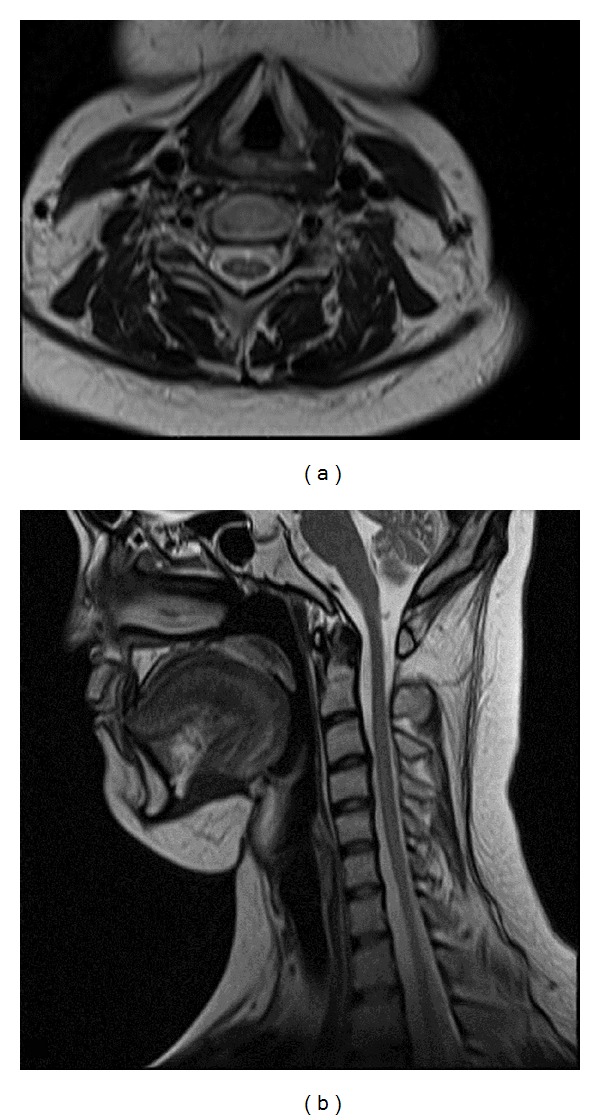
Posttreatment T2-weighted axial (a) and sagittal (b) MR images show normal signal intensity of cervical spinal cord.
